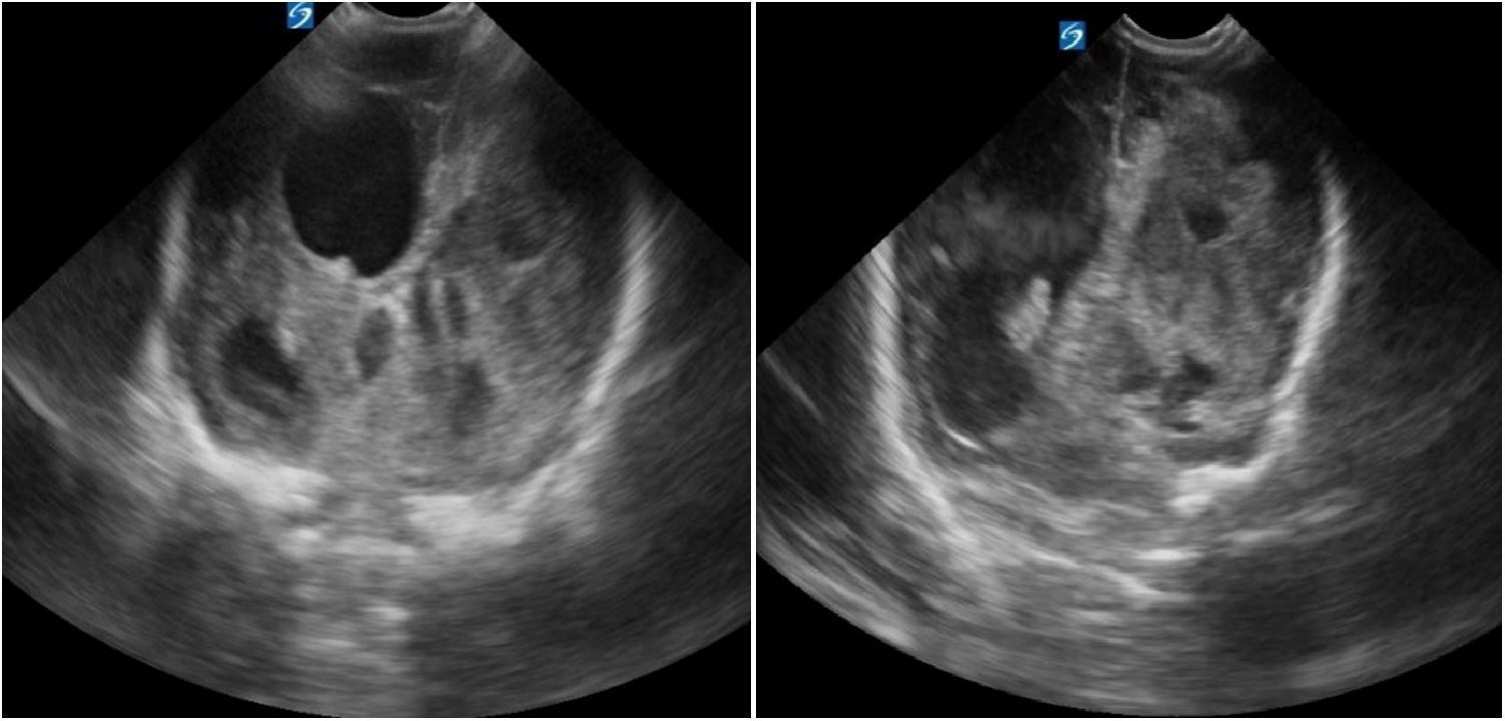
Cranial ultrasound upon admission to our NICU at 22 days of life.
